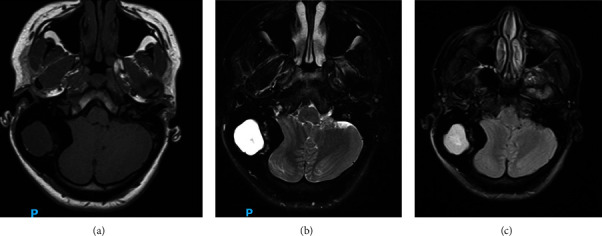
Magnetic resonance imaging in T1 (a), T2 (b), and T2 flair (c) sequencing showing bony expansion and sclerosis of the right temporal bone, fluid within this expansion, and mass effect involving the posterior fossa.
